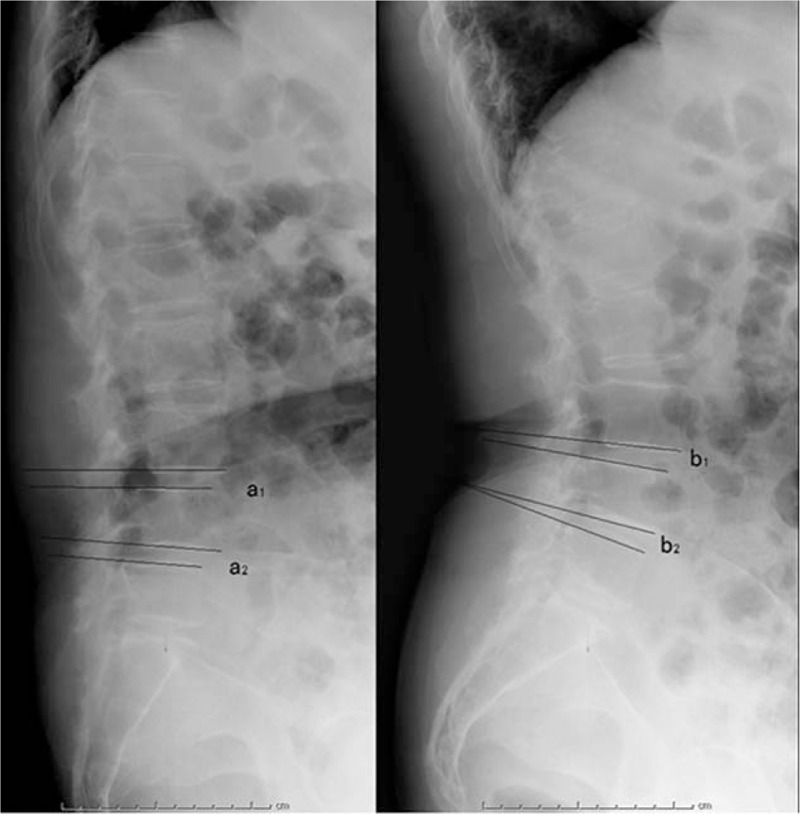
The measurement of the angular displacement on flexion–extension X-ray. The angular displacement of the upper intervertebral space was calculated as (a_1_–b_1_); the angular displacement of lower intervertebral space was determined by calculating (a_2_–b_2_).
